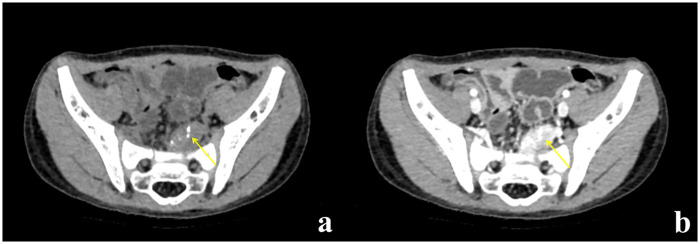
Pelvis CT scan. (a) CT scan showed a mass beside the left internal iliac vessel. (b) Enhancement could be seen on contrast-enhanced CT scan. The yellow arrow indicated the mass.
